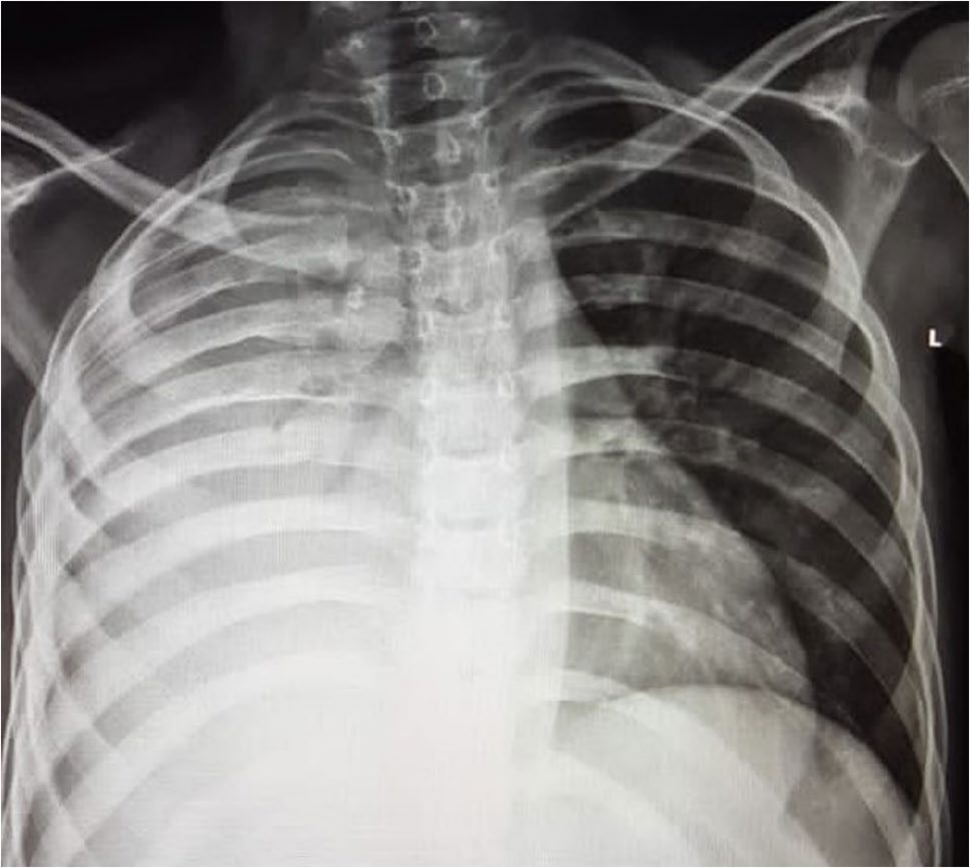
Posteroanterior chest radiograph in a 9-year-old girl with confirmed pulmonary tuberculosis shows a large right-sided pleural effusion with minimum mediastinal shift due to underlying atelectasis
